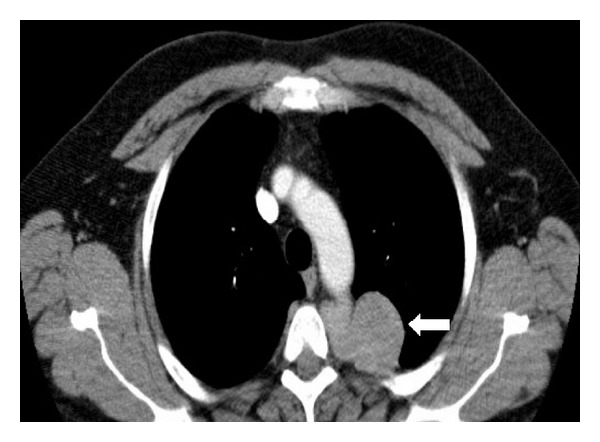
CT thorax with intravenous contrast in a 50-year-old male patient with Castleman disease. A 5 cm well-circumscribed, lobulated mass lesion with homogenously intense contrast enhancement is seen in the left posterior pleural space in a paraspinal location (arrow).
